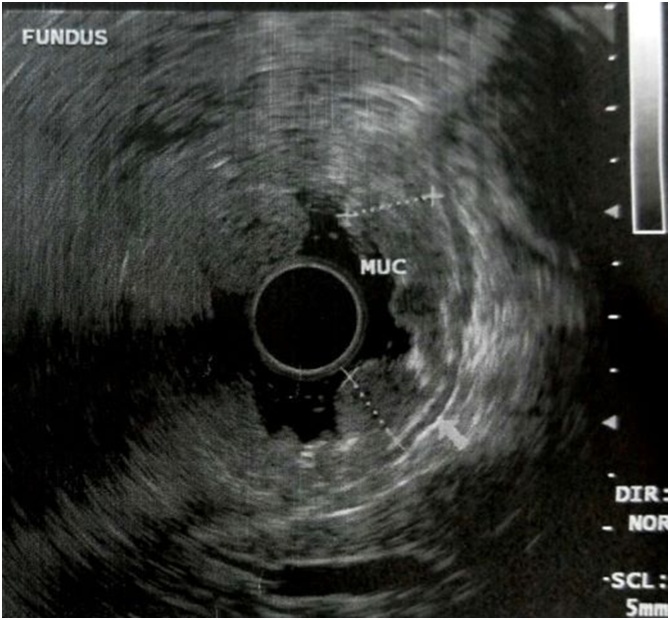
Endoscopic ultrasound from the fundus of the stomach.
